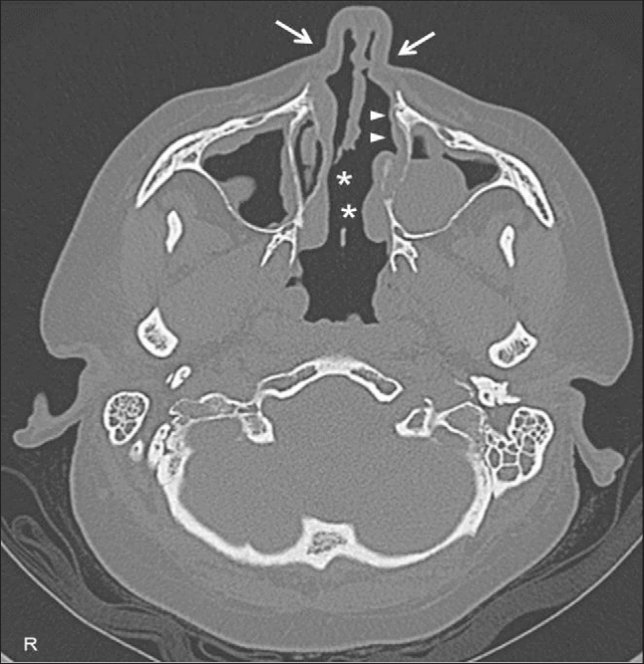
High-resolution computed tomography of paranasal sinuses, axial projection, showing a perforation of the nasal septum (asterisks), the absence of the anterior half of the left inferior turbinate (white arrows), and the atrophy of the upper lateral cartilages (white arrowheads).
